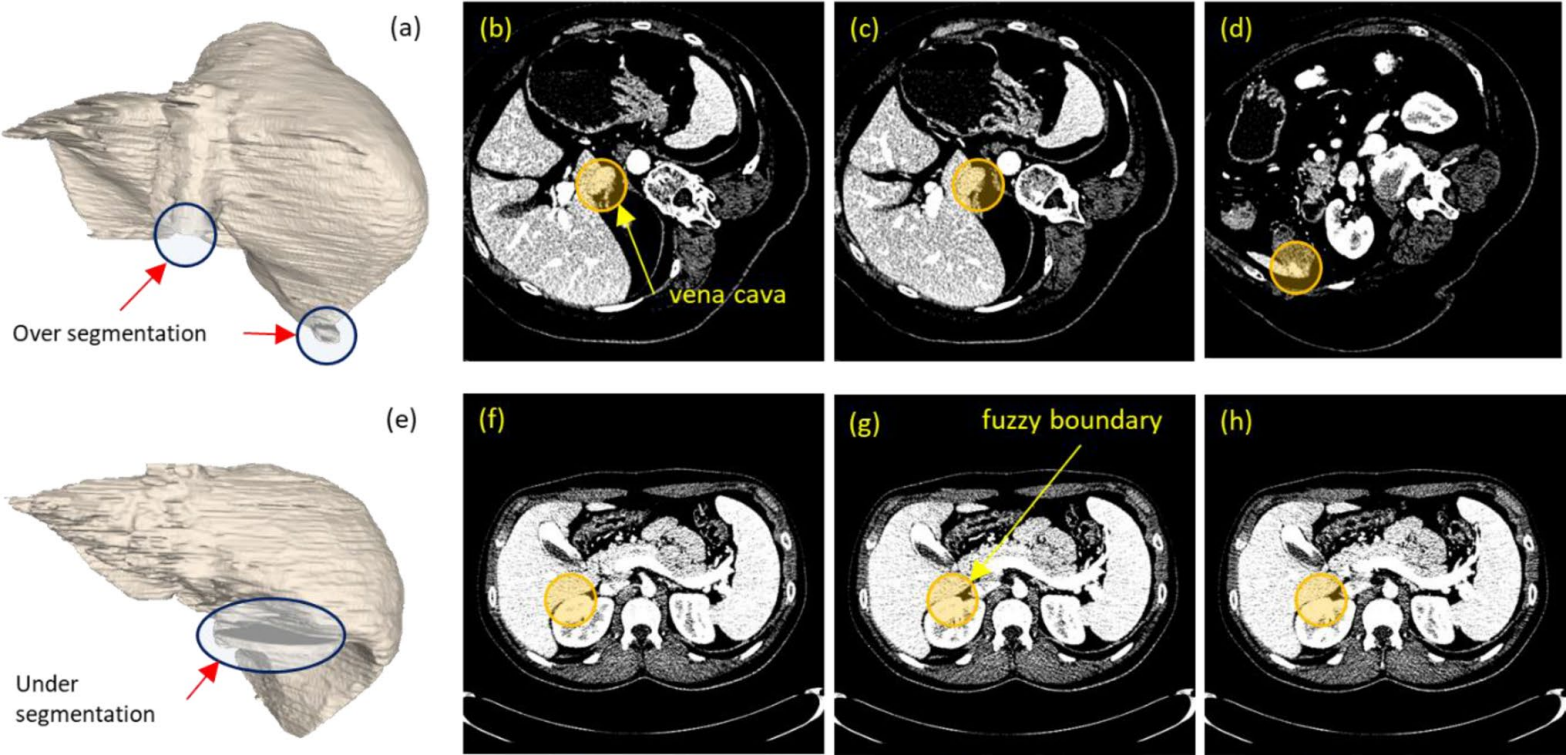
Examples of 3D segmentations (a) and (b), i.e., case 05 (top row) and 09 (bottom row), whose scores were the highest and the lowest, respectively. The corresponding images on their right shows CT slices where over (b), (c), and (d) and under (f), (g), and (h) segmentation occurred.
